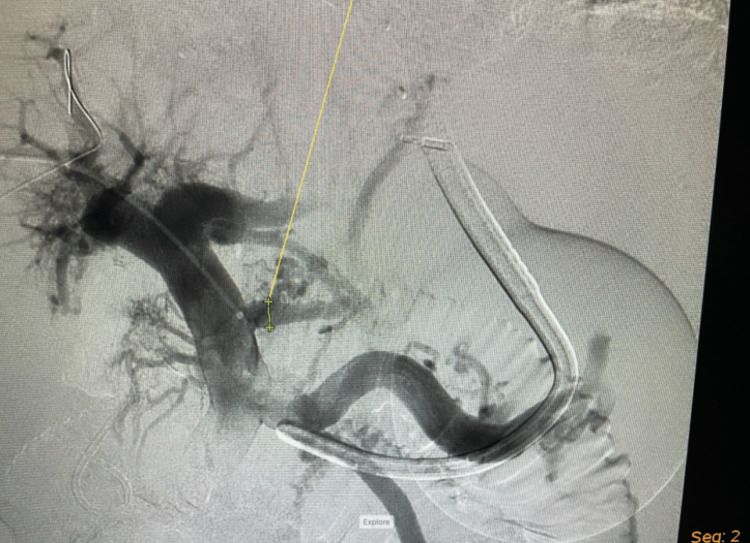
Interventional radiology angiography of patient’s portal circulation and varices with the gastric balloon of the Minnesota tube visible, with overlying gastric varices. You can visualize the appendage distal to the gastric suction port in this radiographic image.
